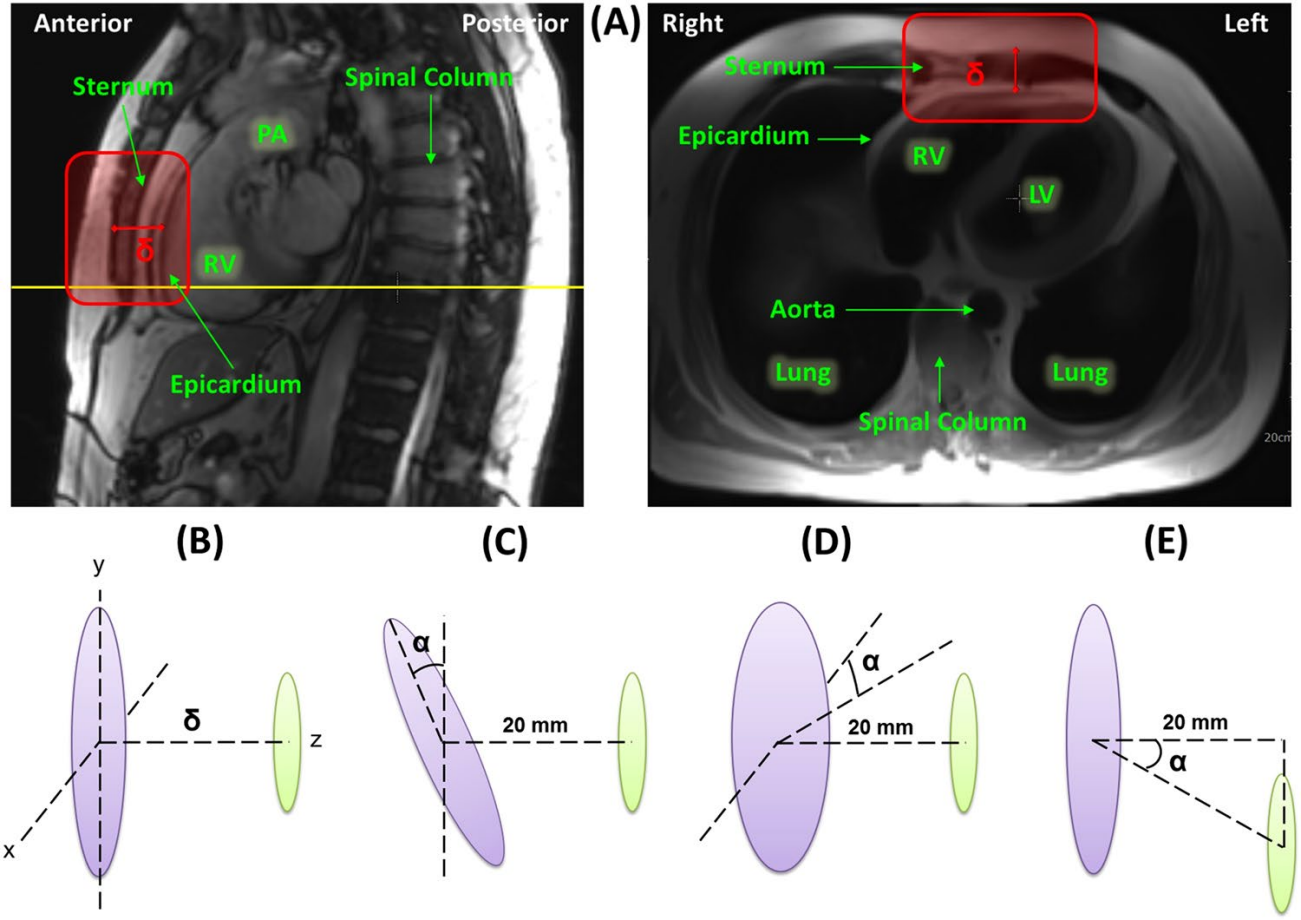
Determining Range of Function. (A) Thoracic MR images in which the left image shows the sagittal view with the yellow line representing the cross-reference line for the axial black blood image in right image. The red box represents the region in which measurements were made in both views. The distance, δ, represents the displacement measured along the region of the red box in which the receiver will be located in the anterior cardiac vein and the transmitter will be located in the anterior chest wall below the adipose tissue along the sternal border. PA: pulmonary artery, RV: right ventricle, LA: left ventricle. (B) Antenna assessment at distance, δ, (C) Antenna assessment with x-axis angular misalignment at angle, α, (D) Antenna assessment with y-axis angular misalignment at angle, α, and (E) Antenna assessment with displacement misalignment at angle, α.
